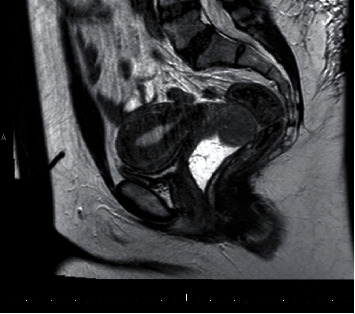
Sagittal T2 MRI of the uterus and cervix with apical vaginal mass in gel-distended vagina.
